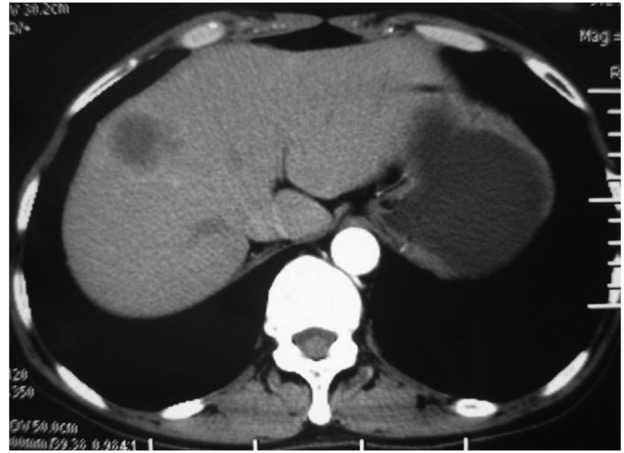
Abdominal computed tomography (CT) showing a hypodense lesion in the right lobe of the liver.
